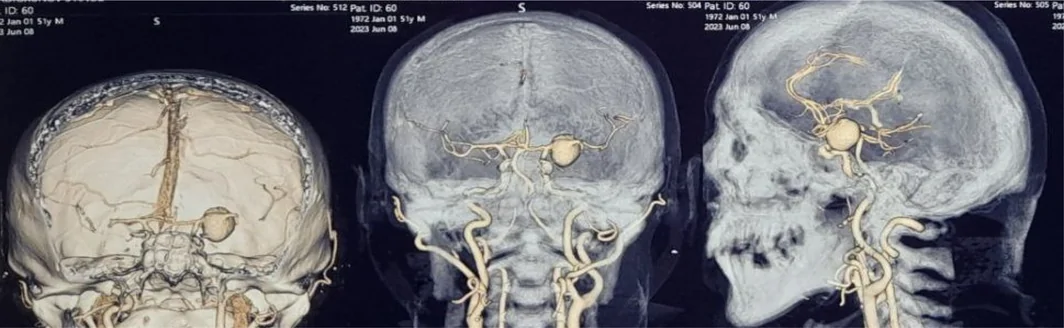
Cranial CT angiography of the cerebral vessels: Axial (first), coronal (middle), and sagittal (last) reconstructions demonstrating a giant left MCA aneurysm. The three orthogonal reconstructions display the fusiform morphology and the giant dimensions of the lesion, together with its continuity with the left middle cerebral artery. The contrast‐filled patent lumen is demarcated from the non‐enhancing mural thrombus, confirming the vascular nature of the lesion.
